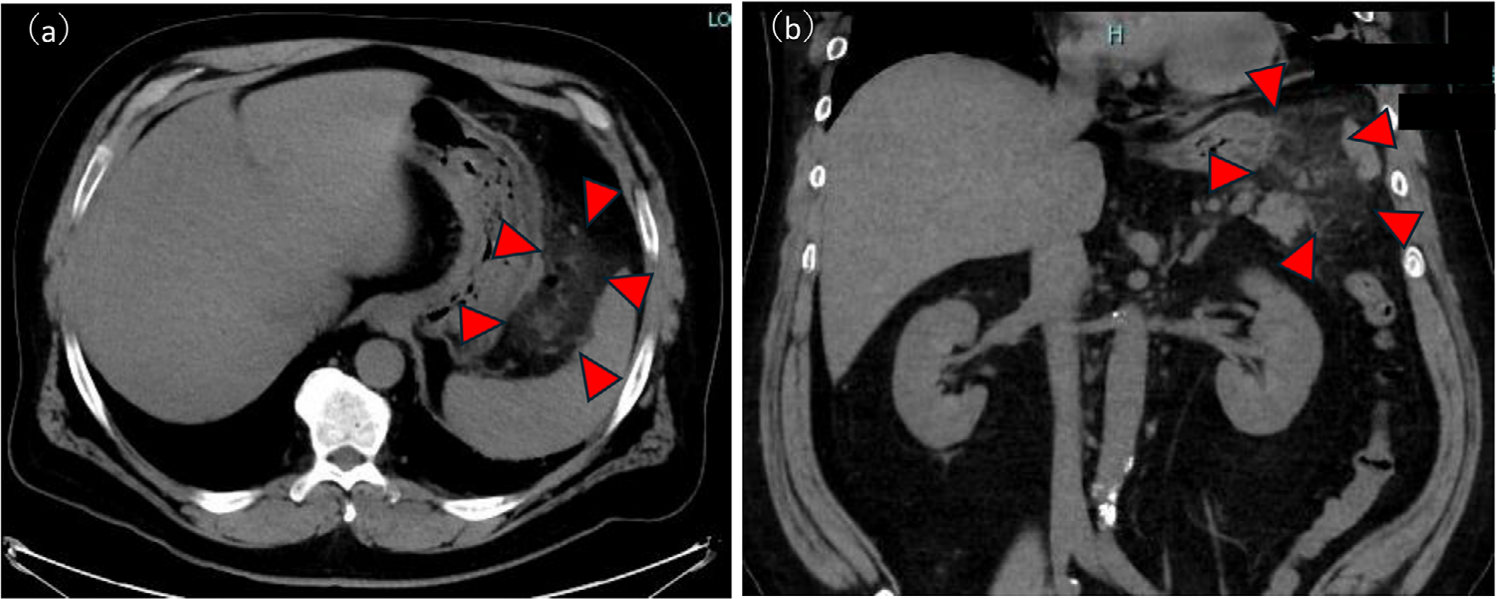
Computed tomography image obtained on postoperative day 4, showing free air and increased fat density around the stomach (red arrowhead), consistent with delayed perforation. (a) Axial view (b) Coronal view.
